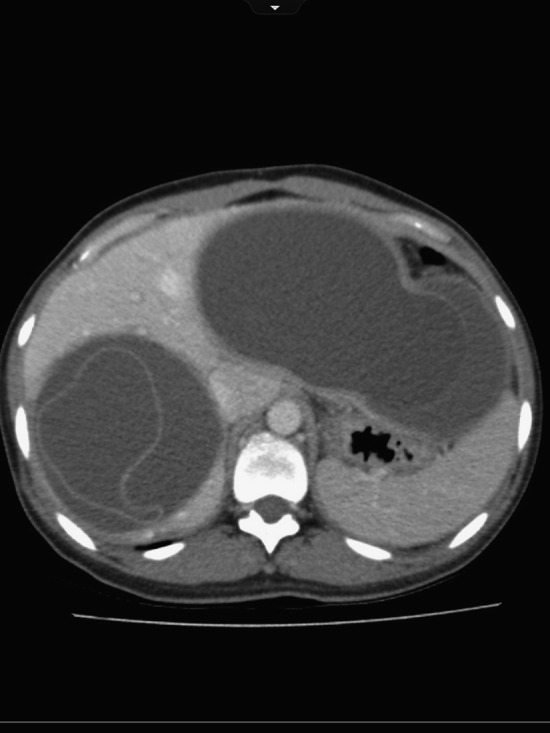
Computed tomography (CT) image of the same two ruptured unilocular hydatid cysts with the clearly visible cyst walls collapsed away from the liver. This is known as the “serpent sign.”
